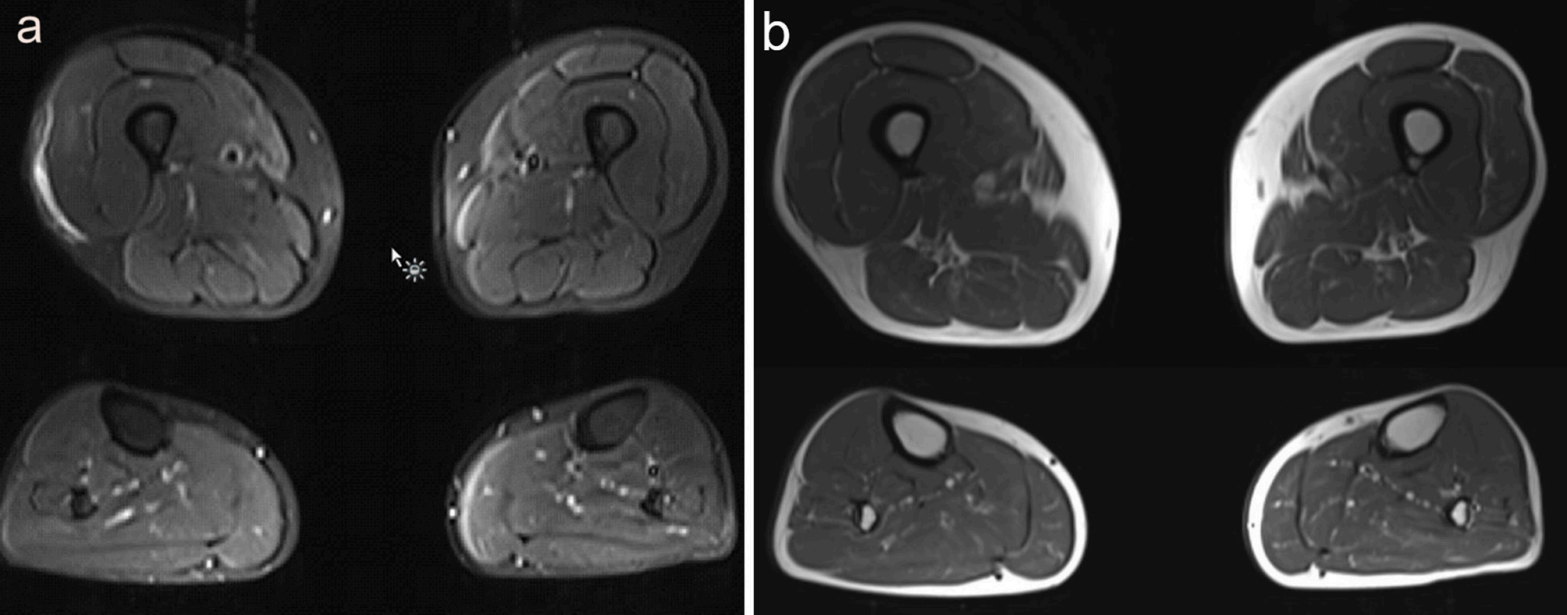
Muscle MRI findings in Case 1. (a) Axial images of STIR MRI sequences through the upper and lower legs show subtle signal change in the right medial thigh, which had been attributed to the patient's muscle biopsy a few days prior. Otherwise, no abnormality was identified on this study. (b) Axial images of T1 MRI sequences through the upper and lower legs show no abnormality.
